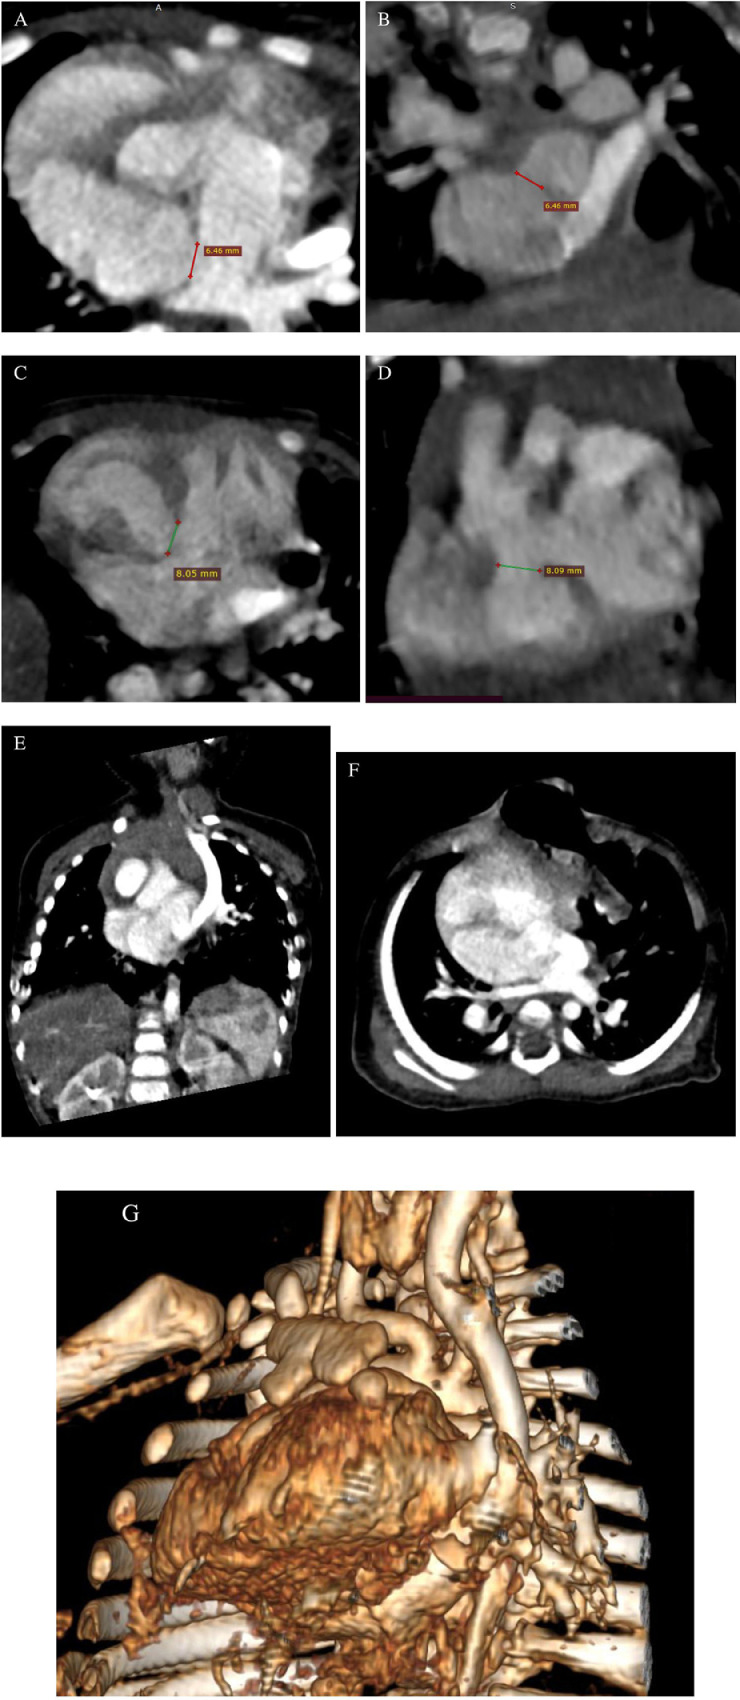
Axial (A) and coronal (B) chest computed tomography (CT) scans of the mediastinal window showed an atrial septal defect. Axial (C) and coronal (D) chest CT scans also revealed a ventricle septal defect. Coronal (E), axial (F), and 3D reconstruction (G) showed a persistent left superior vena cava (PLSVC).
